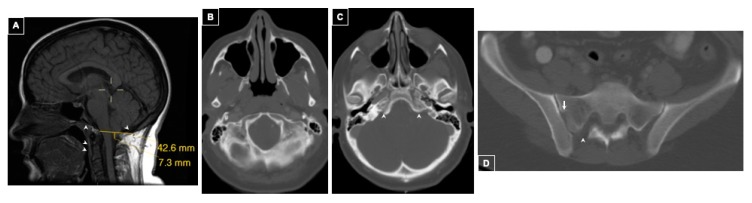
Patient 1 Intracranial and Spinal Imaging. Panel (A): Mid-sagittal T1-weighted sequence shows lowest tonsillar position of 7 mm through the foramen magnum. Narrow underdeveloped clivus and occipital bone as well as uncalcified synchondroses of the odontoid are appreciated (arrowheads). Panel (B): Axial CT of the head revealed abnormal ossification of the occipital bone. Panel (C): Axial CT of the head showing uncalcified petroclival synchondrosis (arrowheads). Panel (D): Axial CT of lumbo-sacral spine shows spina bifida occulta of S1 (arrowhead) and segmentation anomaly of the sacral ala (arrow).
